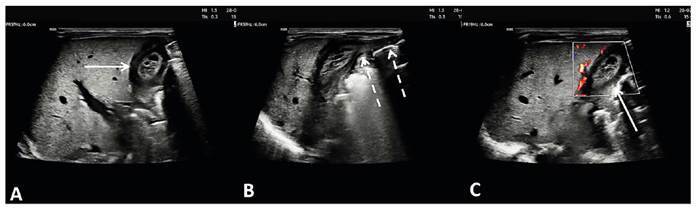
(A-B) Sonographic examination showing a heterogeneous partially solid and cystic tubular mass (arrow) arising from the left lobe of the liver to the umbilicus along the tract of the umbilical vein (interrupted arrow). (C) No flow was detected in the lesion on Doppler US (arrow).
